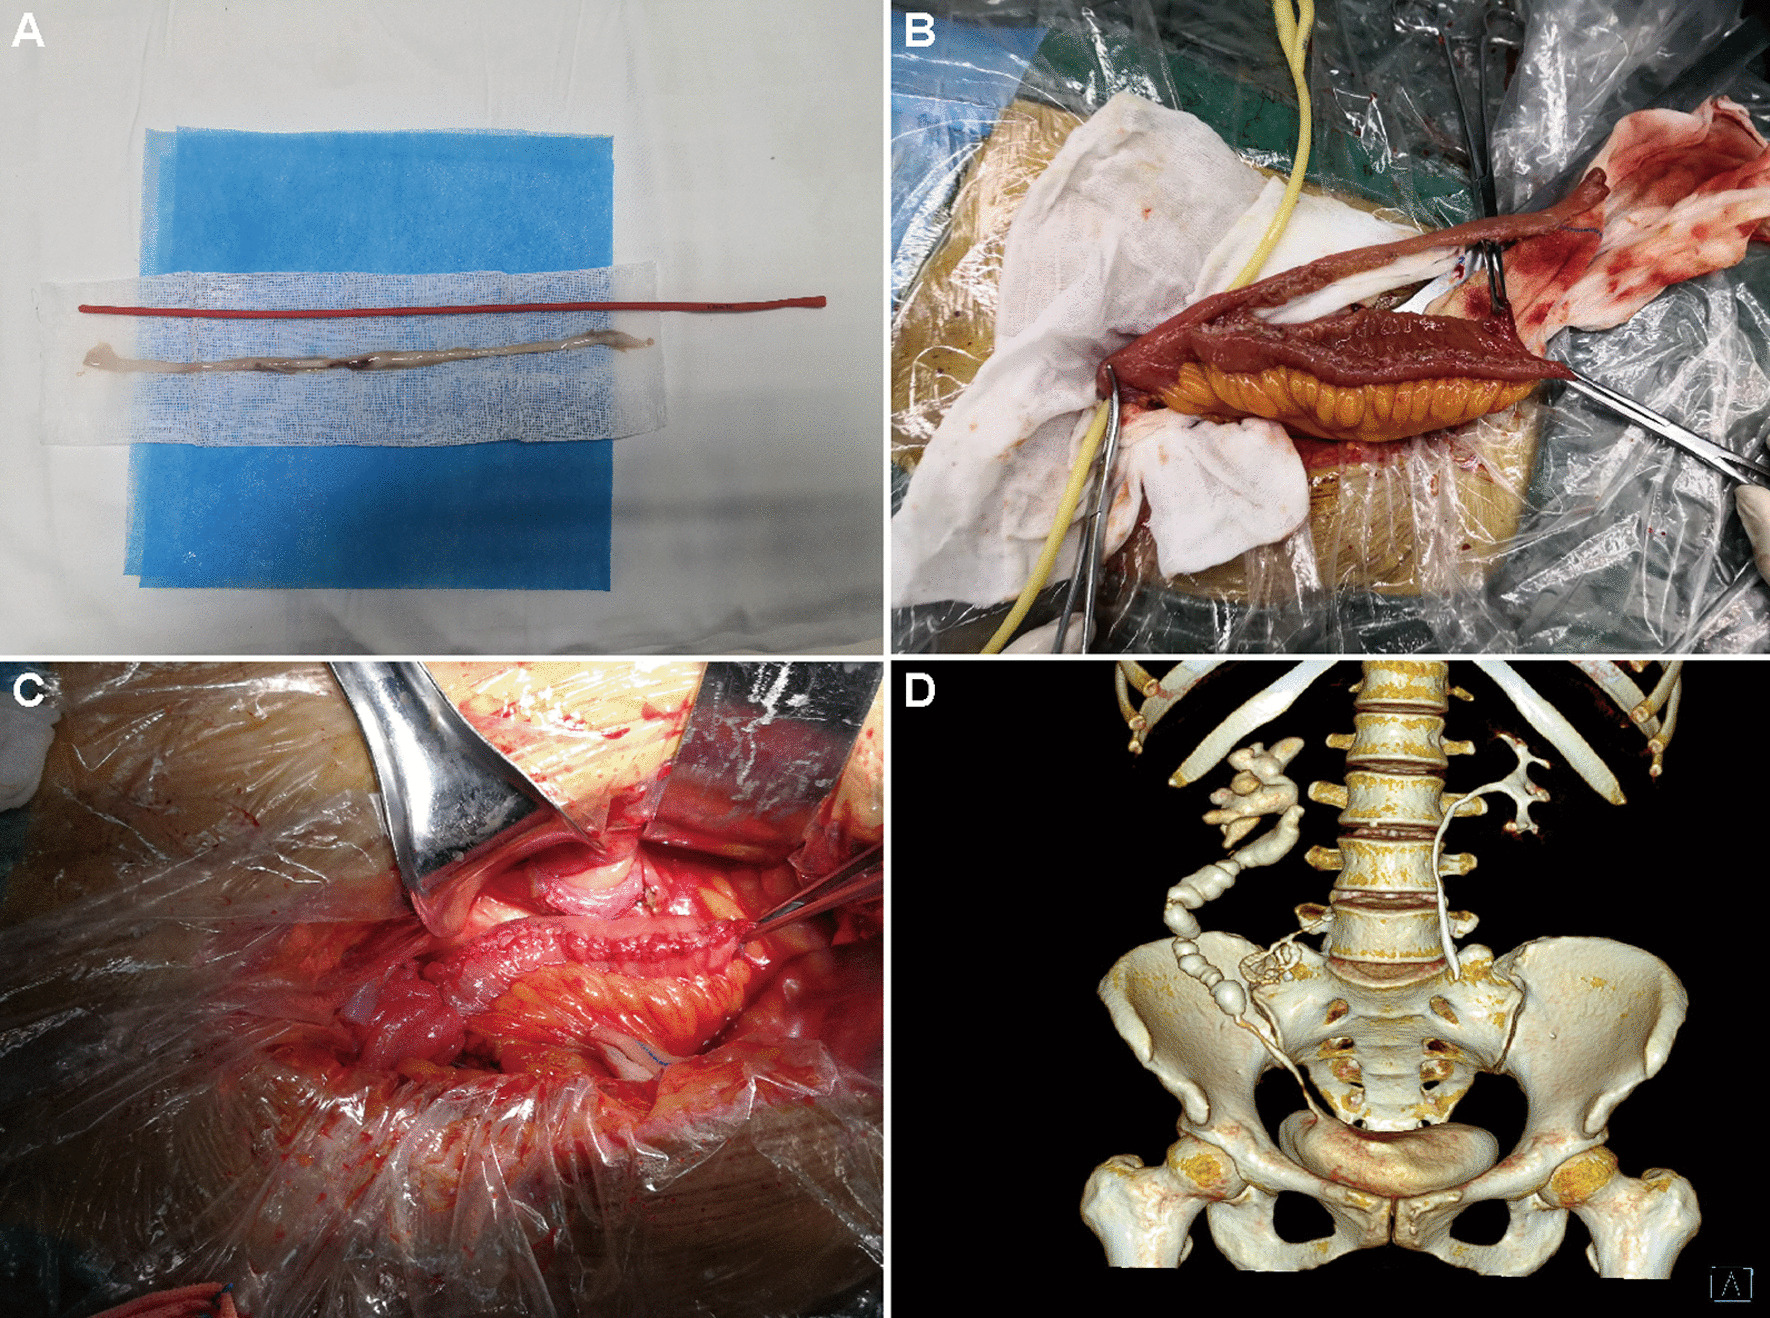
Images of patient 1. A Evacuated panureter approximately 28 cm in length. B Tapering of the isolated ileum. C Bridging the ureter by retubularized TDI. D CT urogram in month 30 post-surgery
